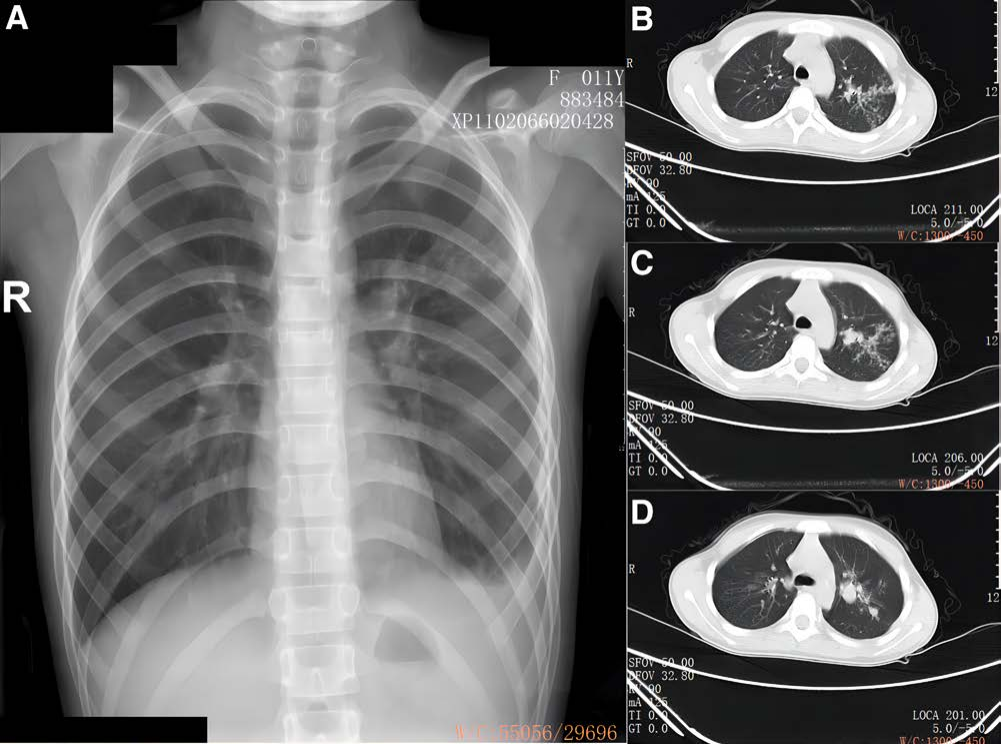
Chest X-ray and chest CT of the patient’s daughter taken on August 30, 2022.
